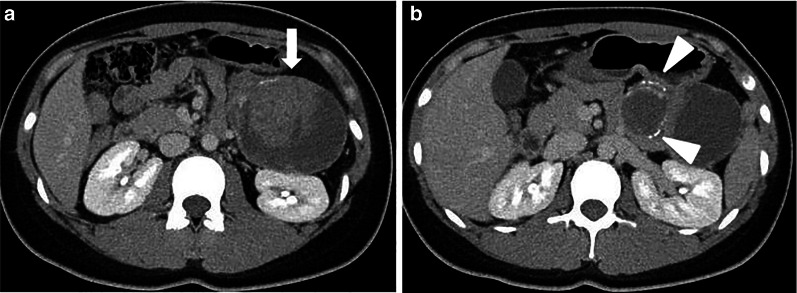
Abdominal computed tomography scan images. A 10-cm clearly demarcated tumor (arrow) with solid and cystic components are seen in the pancreatic tail (a), and the capsule had calcifications (arrowhead) (b)
